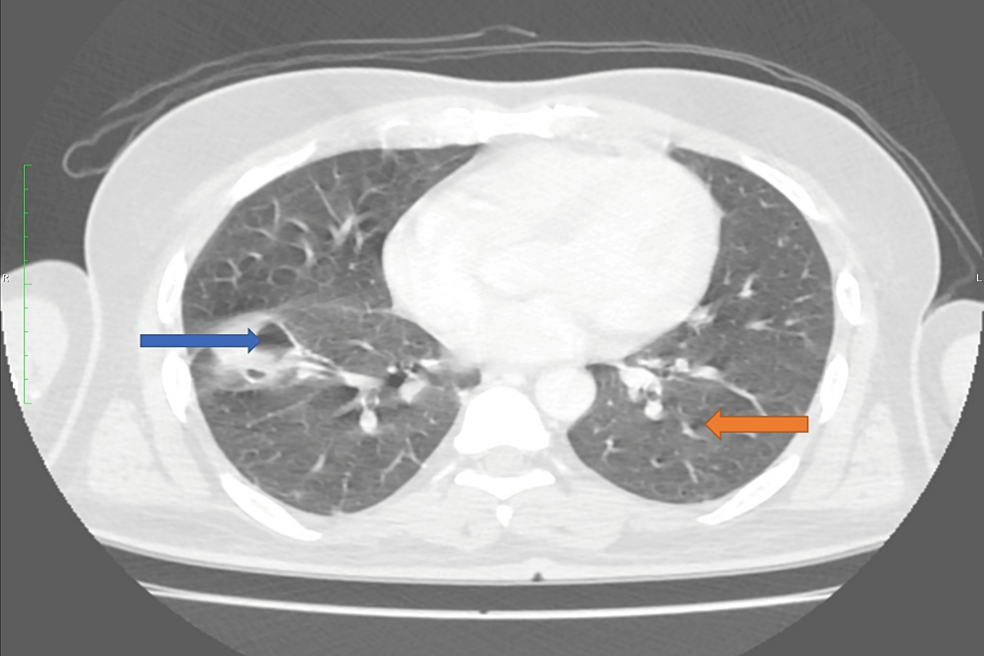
HRCT chest image High-resolution computed tomography (HRCT) chest showing cavitating nodules in bilateral lung fields, the largest measuring 3.9 x 2.5 cm in the antero-basal segment of the right lobe (shown in blue arrow).  Multiple small solid nodules were noted in bilateral lung fields, the largest measuring 7 mm in the superior segment of the left lower lobe (shown in orange arrow).
